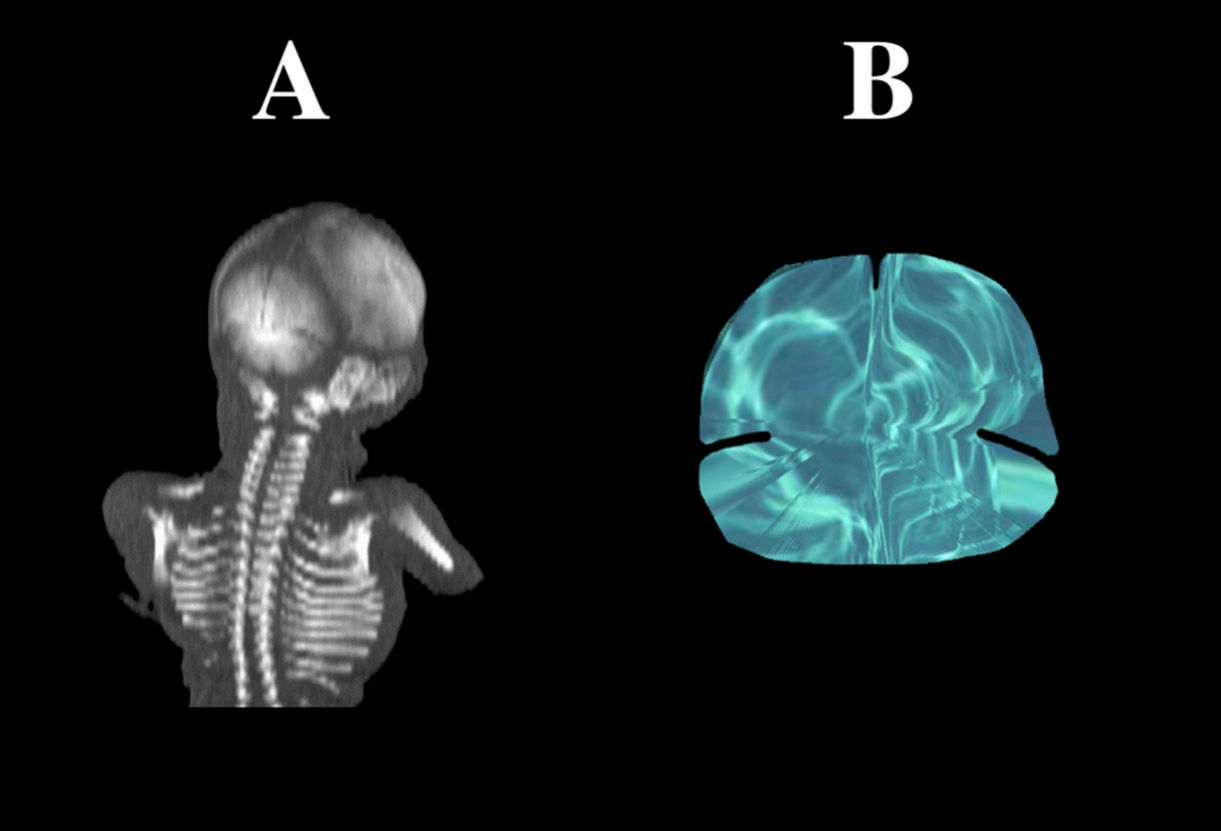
A male human fetus aged 20 weeks on frontal projection (A), 3D reconstruction of the fused occipital squama ossification center (B) using Osirix 3.9 MD. The gray scale of the obtained CT images expressed in Hounsfield units (HU) varied from −275 to −134 for minimum, and from +1165 to +1558 for maximum. Therefore, the window width (WW) was varied from 1.404 to 1.692, and the window level (WL) varied from +463 to +712. Parameters of the imaging protocol were as follows: mAs: 60, kV: 80, pitch: 0.35, FoV: 180, rot. time: 0.5 sec.; while those of the CT data were as follows: slice thickness: 0.4 mm, image increment: 0.6 mm, and kernel: B45 f-medium.
